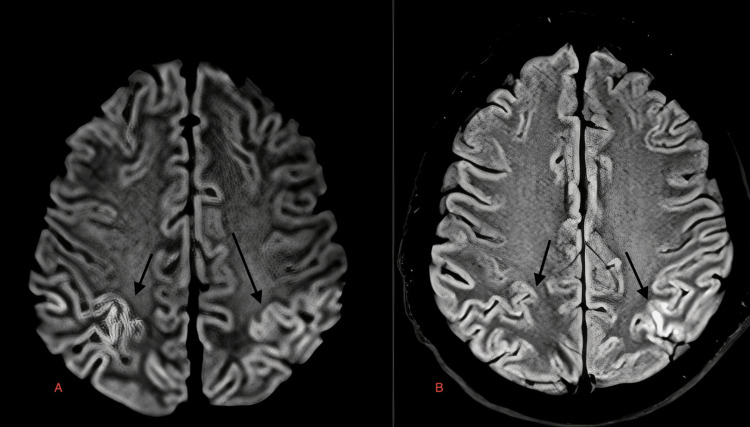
Case 5: Interval increase in the cortical hyperintensities are seen in both parieto-occipital lobe on DWI and FLAIR images (black arrows in A and B, respectively) of a 40-year-old male patient six days after presentation with dengue encephalitis. DWI: diffusion-weighted image; FLAIR: fluid-attenuated inversion recovery sequence
